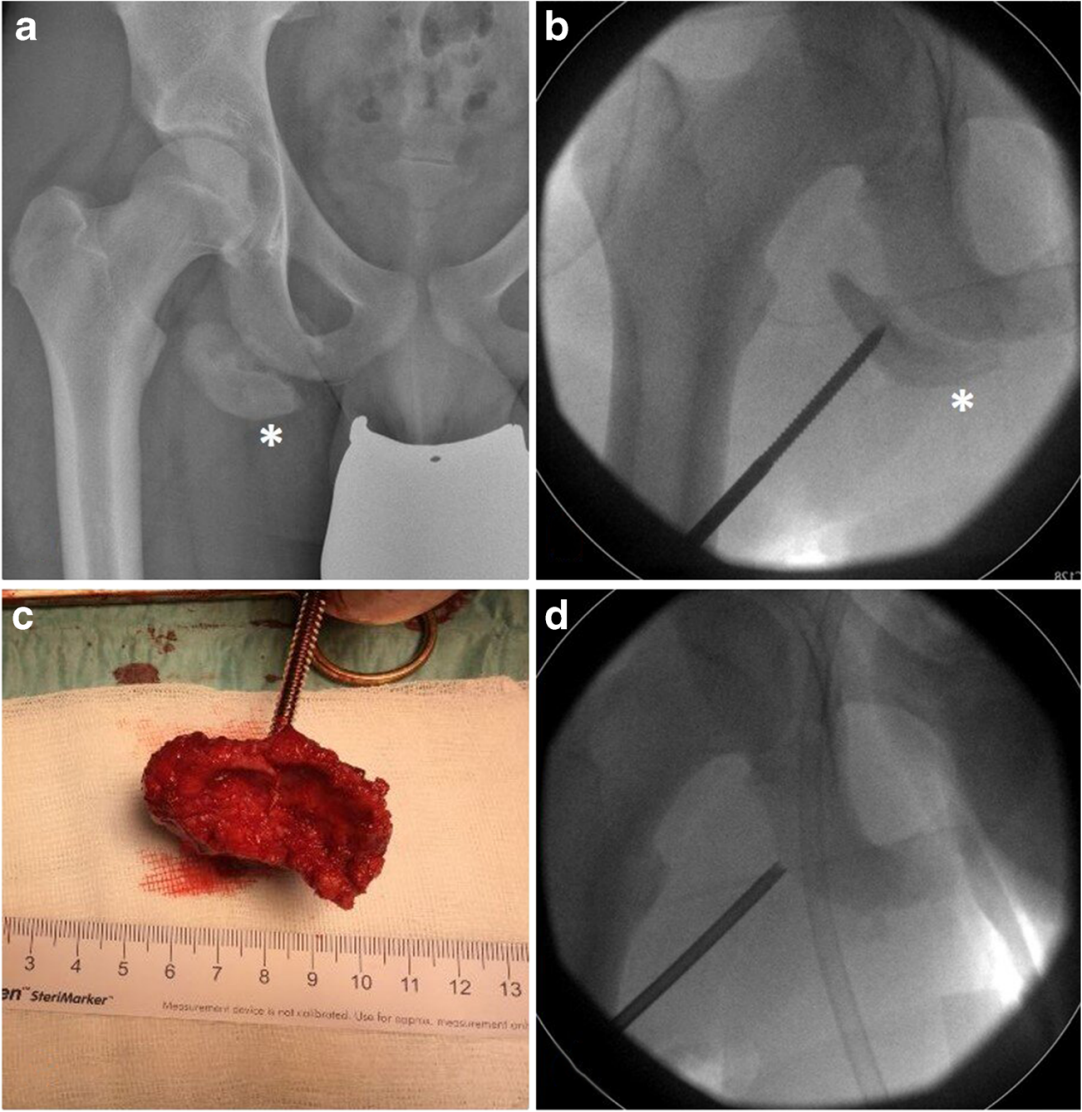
Hypertroph bony fragment in a chronic right-sided avulsion fracture 1 ½ years after trauma (a: plain semipelvis radiograph, b: attempt of anatomic repositioning of dissected bony fragment, c: excised bony fragment, d: intraoperative control of subsequent anchor placement for tendon refixation, star*: bony fragment)
